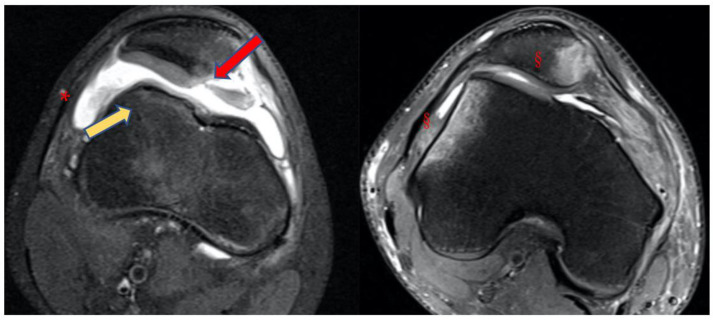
Case of a primary patellar dislocation, shown in MRI axial views. Note the large amount of synovial fluid or hemarthrosis (*), the bone bruise on its typical presentation (§), the chondral defect on the medial side of the patella (red arrow), and the shape of the trochlea (yellow arrow).
